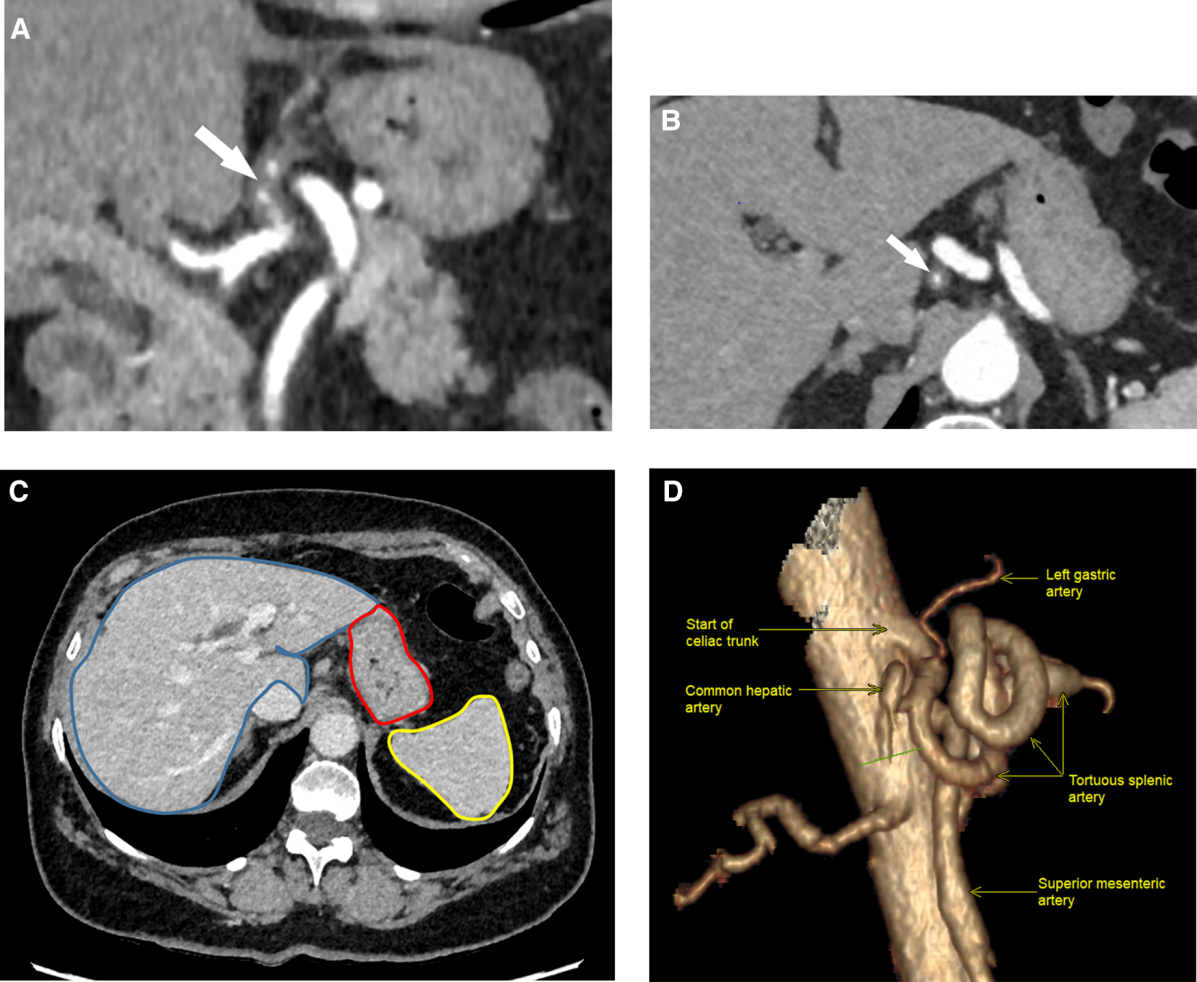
Helicoidal 512 slice computed tomography angiography in arterial phase with 1.3 mm slices. (A) Sagittal view showing eccentric wall thickening of the LGA (arrow) from its start of the coeliac artery, evoking a dissection. (B) Coronal view of LGA dissection showing a diffuse irregular luminal narrowing (arrow). LGA, left gastric artery. (C) Normal perfusion of gastric wall (red mark), liver (blue mark) and spleen (yellow mark) in portal phase. (D) 3D-reconstruction of the celiac trunk anatomy (right lateral view, 90°).
